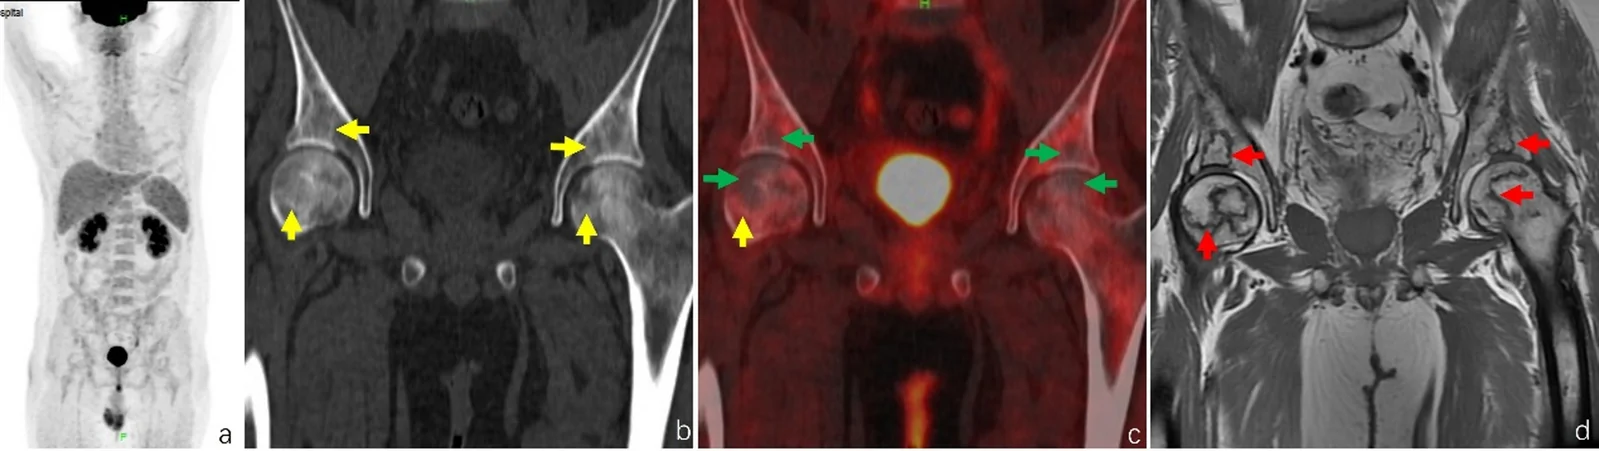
Osteonecrosis of the femoral heads and iliac bones. PET/CT and MRI images from a 25-year-old male (No. 7) with T-lymphoblastic lymphoma after allogeneic hematopoietic stem cell transplantation. The maximum intensity projection (a) and CT image (b) demonstrate multiple linear hyperdense lesions (yellow arrows) within the bilateral femoral heads and iliac bones. PET/CT fused image (c) reveals decreased ^18^F-FDG uptake (green arrows) with peripheral increased uptake (yellow arrow). An MRI scan performed the day after the PET/CT shows multiple geographic abnormal signals (red arrows) at the bilateral femoral heads and iliac bones on the T1-weighted image (d). According to the modified 2019 version of the Association Research Circulation Osseous international classification of osteonecrosis staging system, the osteonecrotic lesions of the bilateral femoral head are classified as stage 2
